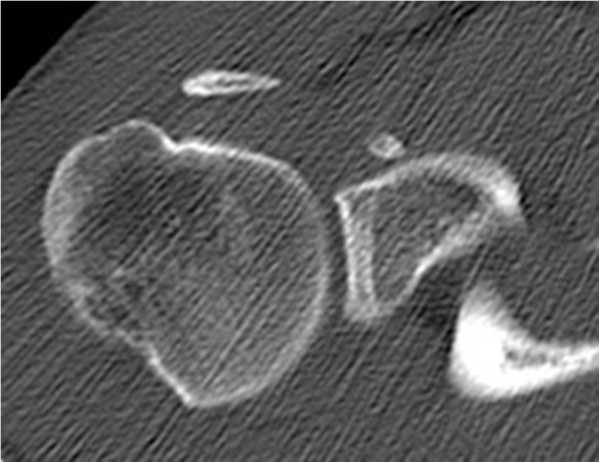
Preoperative computed tomography scan showing the osseous piece.
